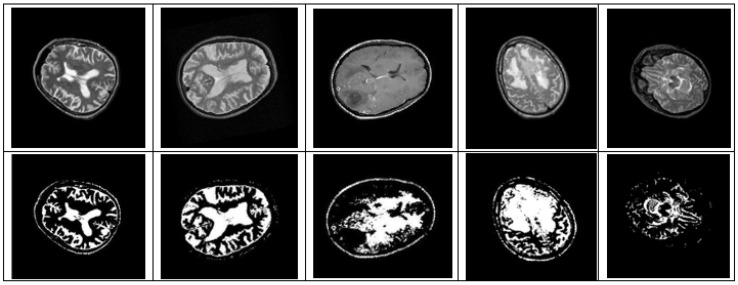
Rotation Invariant (−180° to +180°) Brain MRI Image Detected by CNN.
